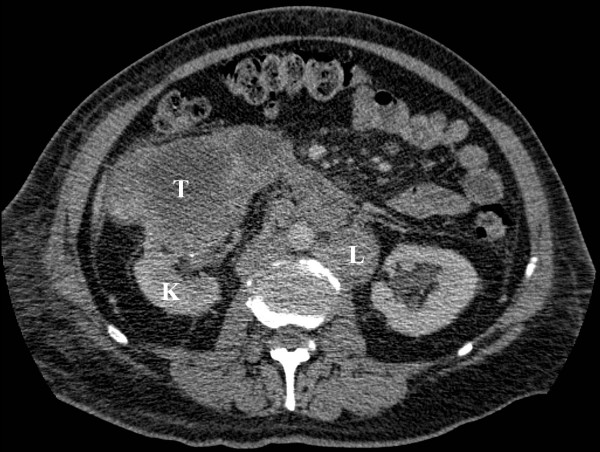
Axial CT through the mid-abdomen following intravenous contrast: the normal part of the right kidney(K) is seen posteriorly with a large mass containing central necrosis arising anteriorly(T). Enlarged lymph nodes(L) are also seen adjacent to the aorta.
